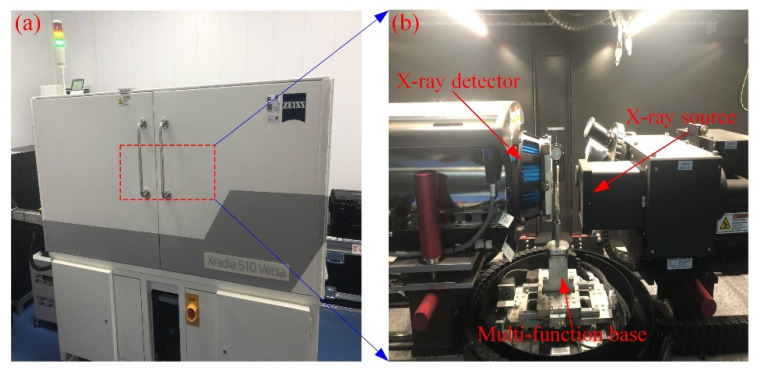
Zeiss Xradia 510 Versa high-resolution CT system (a) and interior (b).
